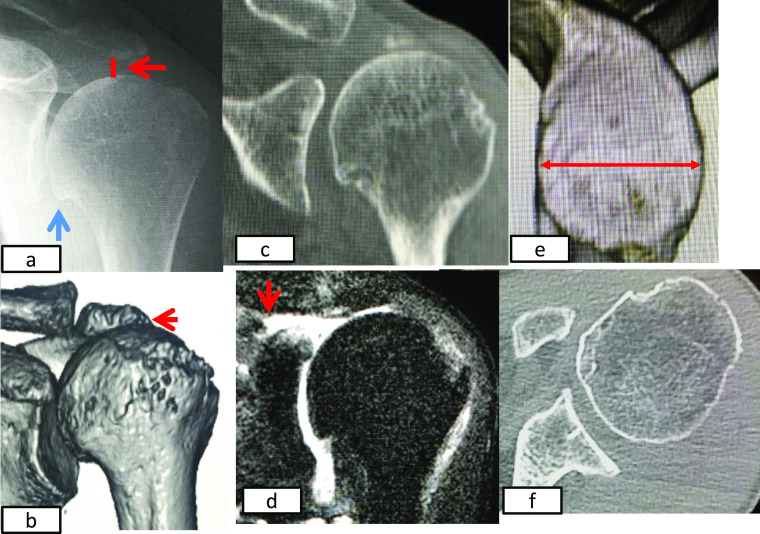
Preoperative images. a) Plain radiography shows an acromio-humeral head interval (AHI) of 2 mm
